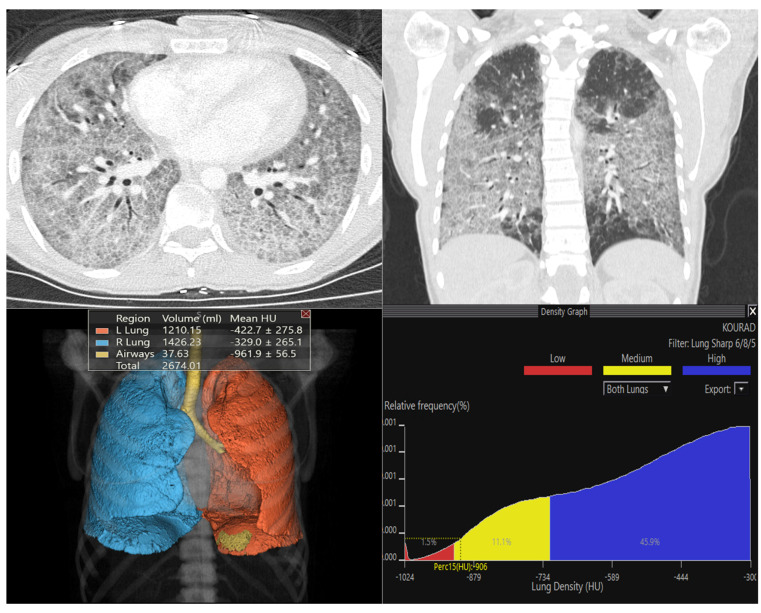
Quantitative analysis of a case presenting with bilateral symmetric high-density parenchymal involvement. The upper panel reveals diffuse, symmetric ground-glass opacities and consolidation areas in both lungs on axial and coronal CT sections. The volumetric analysis in the lower left quadrant demonstrates a marked increase in mean parenchymal density (right: −329 HU; left: −422 HU). The histogram analysis in the lower-right quadrant shows a pathological shift of the density curve to the right (toward higher density). It is observed that normal aerated lung tissue has been largely replaced by high-attenuation (consolidated) areas, represented by the blue zone in the graph, which accounts for 45.9% of the total volume.
